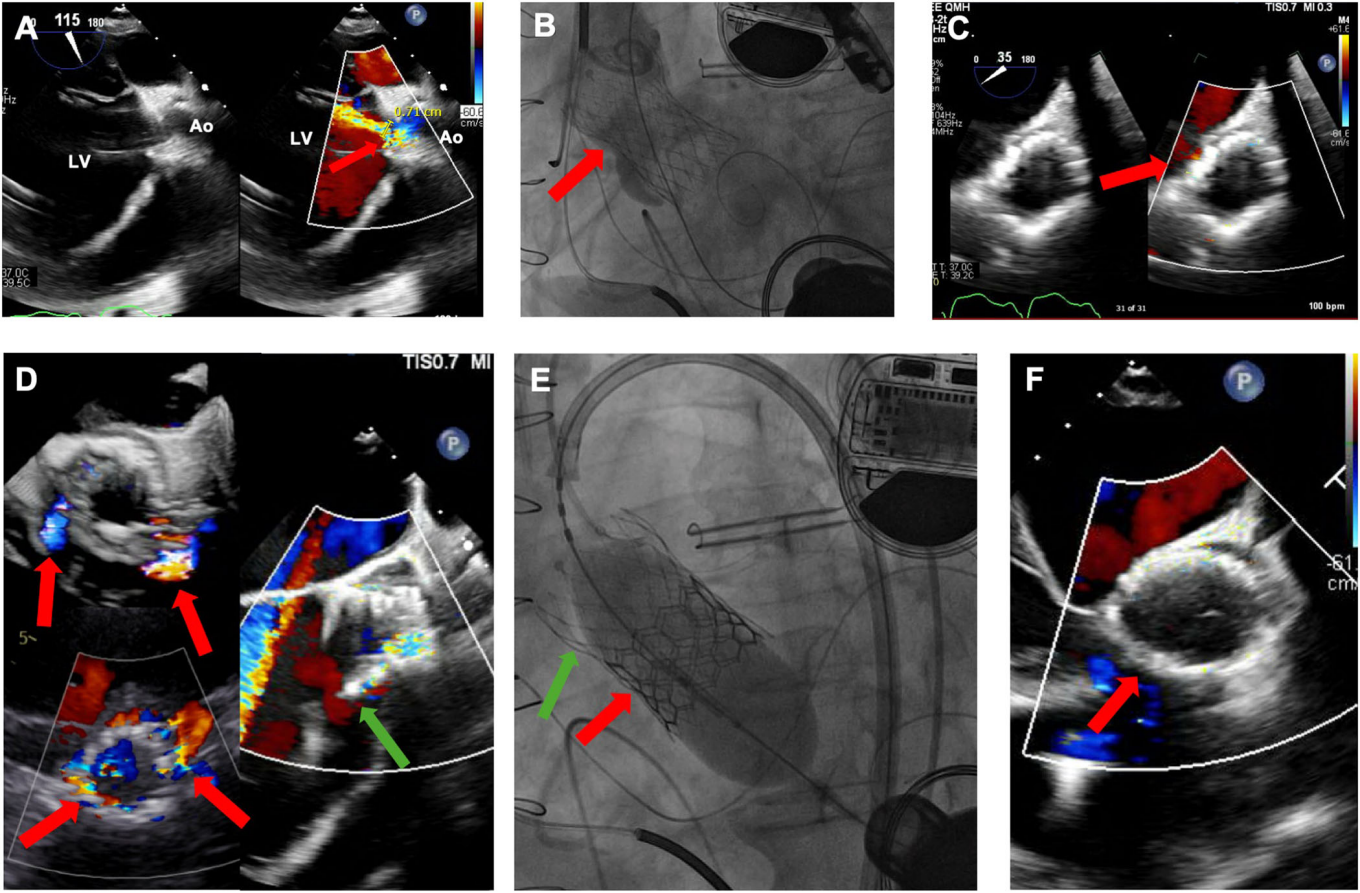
Patient 1. (A) Severe aortic regurgitation with vena contracta (VC) 0.7 cm [red arrow] echocardiography. (B) Successful Evolut R deployment confirmed on fluoroscopy [red—arrow Evolut R]. (C) Trivial AR following Evolut R deployment. (D) Severe paravalvular leak of Evolut R THV with downward migration of Evolut R THV toward left ventricular side [red arrow—paravalvular leak; green arrow—downward migration of Evolut R THV toward left ventricular side]. (E) Valve‐in‐valve TAVI with deployment of Edwards Sapien 3 THV within Evolut R THV [red arrow—Edwards Sapien 3; green arrow—Evolut R THV]. (F) Echocardiography showing trivial residual AR [red arrow—BEdwards Sapien3 within Evolut R]. [Color figure can be viewed at wileyonlinelibrary.com]
